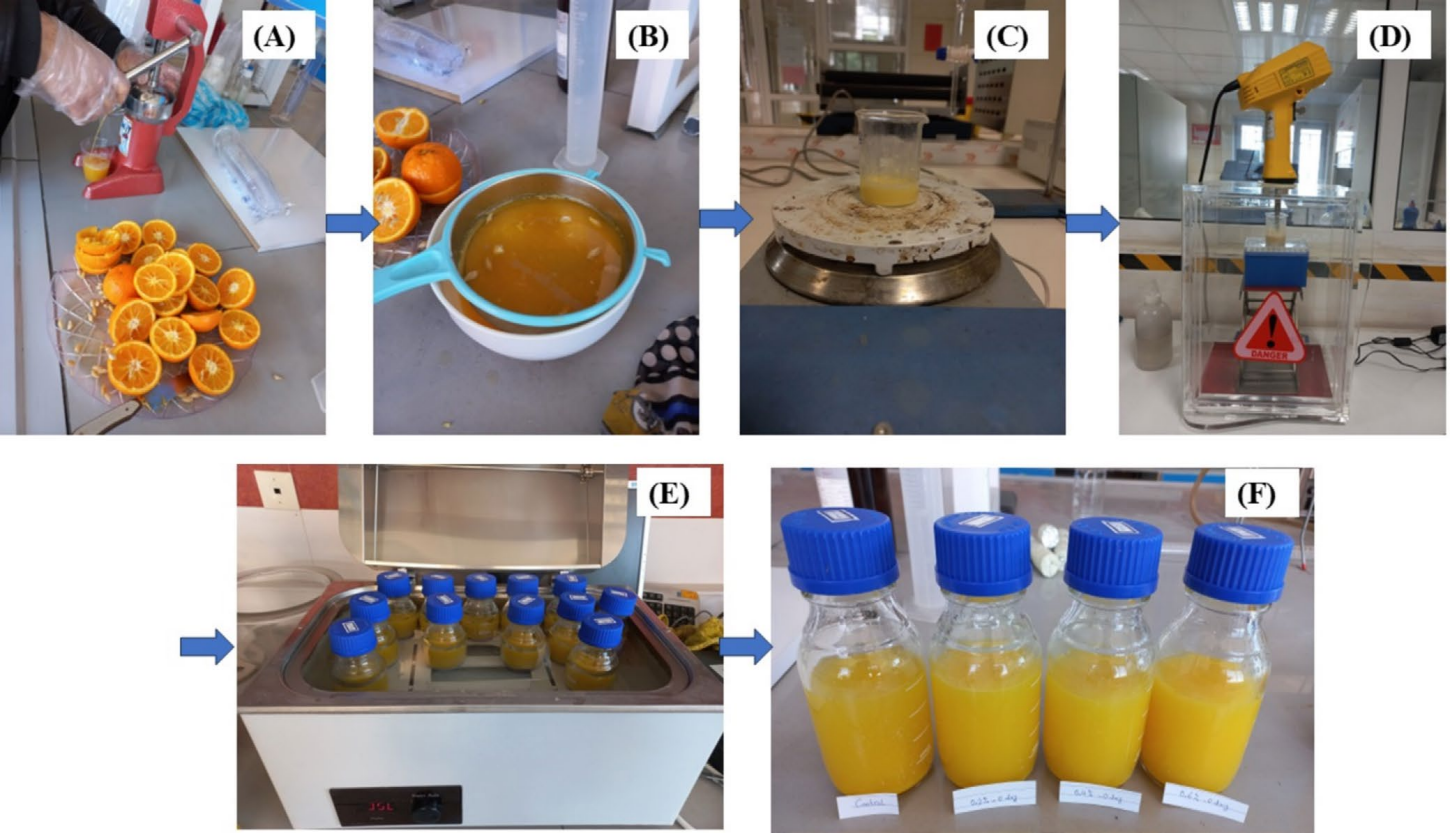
Preparation of orange juice containing LGEO nanoemulsion (A) juicing oranges, (B) filtering the orange juice, (C) mixing the orange juice with LGEO nanoemulsion, (D) ultrasonication of samples, (E) pasteurization of samples at water bath, and (F) prepared orange juice samples.
